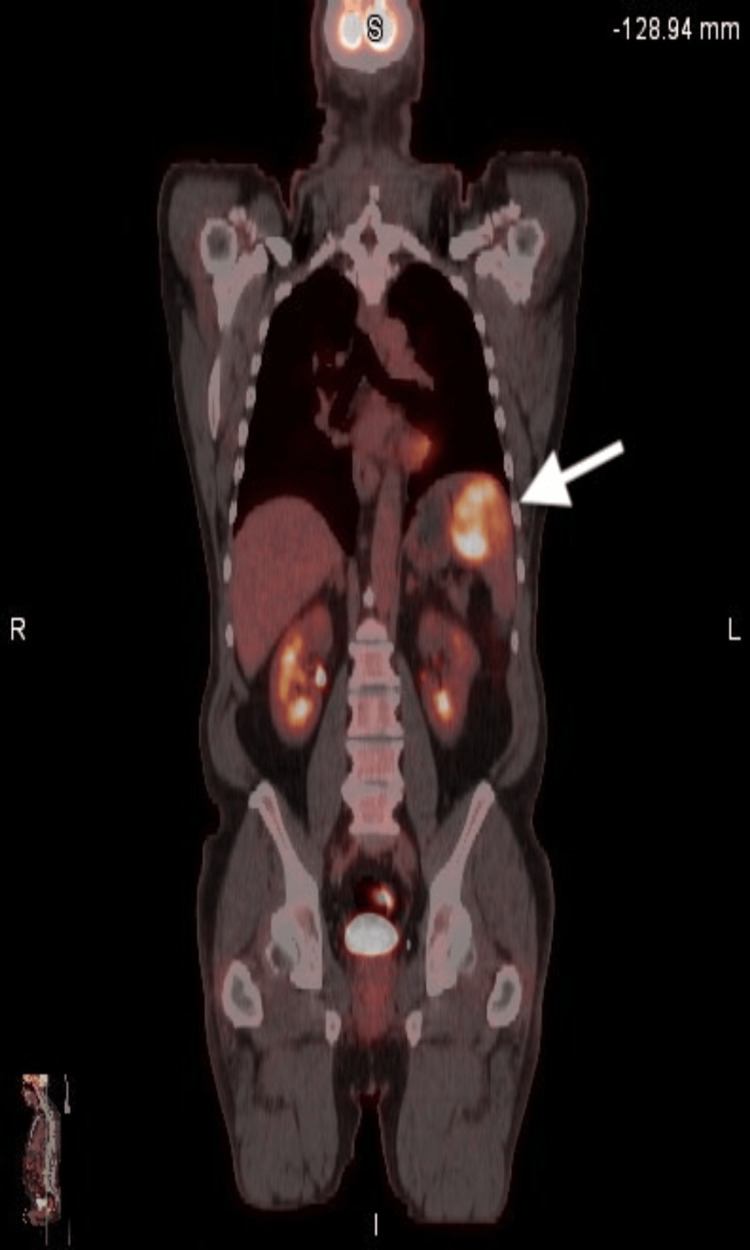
Coronal section of PET-CT scan showing solitary FDG-avid splenic mass (arrow) PET-CT, positron emission tomography-computed tomography; FDG, fluorodeoxyglucose
